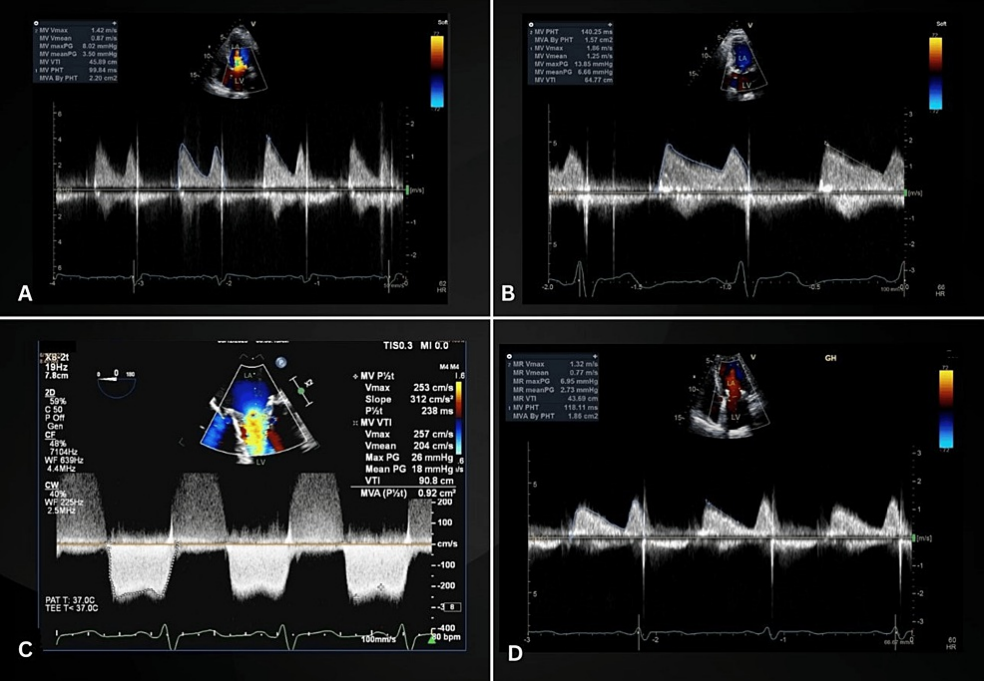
TTE and TEE detailing bioprosthetic valve hemodynamics (A). Baseline TTE obtained in 2018 demonstrating normal bioprosthetic valve hemodynamic. (B) TTE from January 2023 with a prolonged PHT and elevated mean transvalvular gradient. (C) TEE from June 2023 revealing further prolongation of the PHT and a marked elevation in mean PG. (D) TTE from July 2023, post-anticoagulation therapy with apixaban, revealing a complete reversal of the mentioned hemodynamic parameters to normal values TTE: transthoracic echocardiogram; TEE: transesophageal echocardiogram; PHT: pressure half-time; PG: pressure gradient; LA: left atrium; LV: left ventricle
